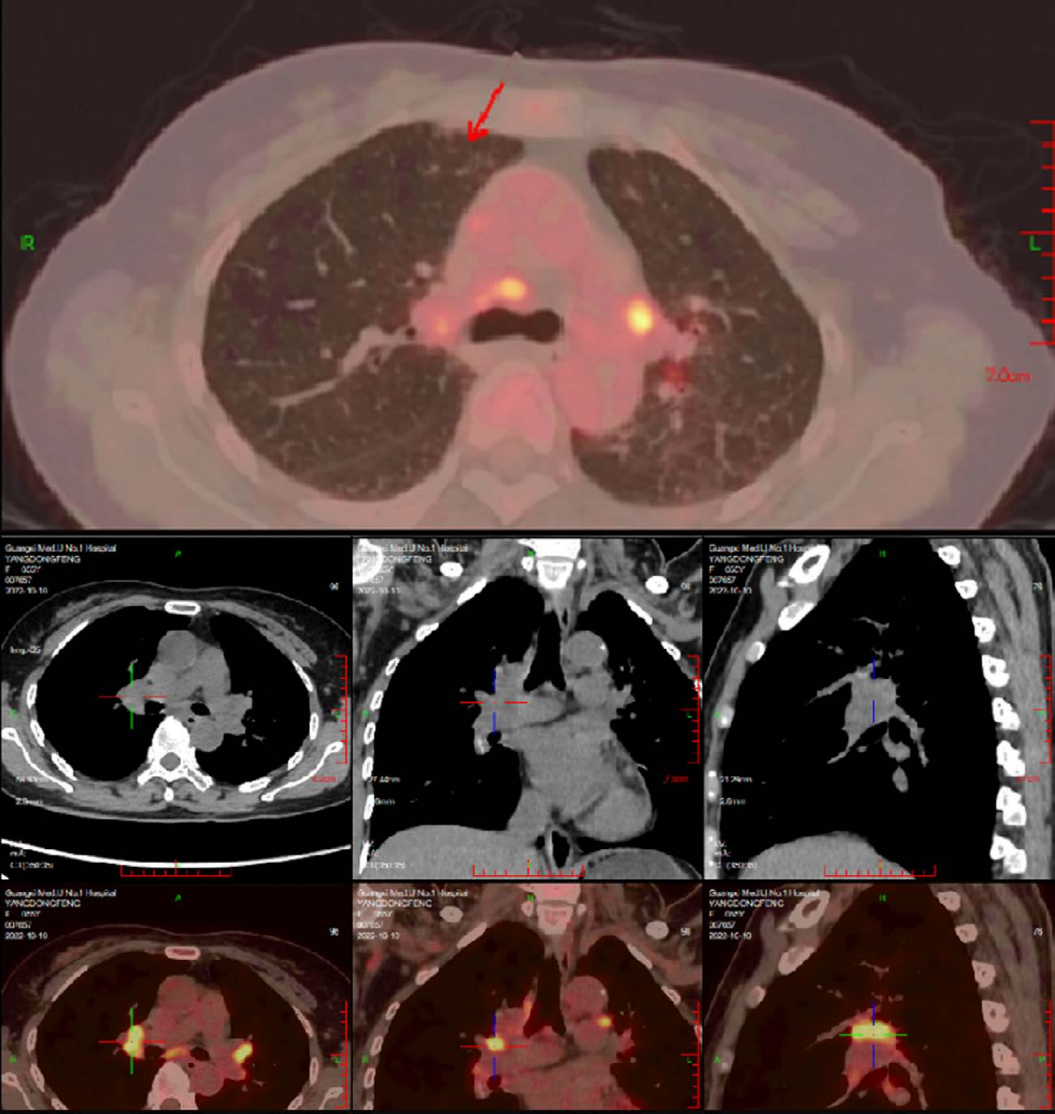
PET/CT images of the patient. Arrows showed inflammatory hyperplasia of multiple systemic lymph nodes (bilateral neck areas I, II, and V, bilateral axillae, mediastinum, bilateral hilum, and bilateral groin) and a slight inflammation in both lungs. PET/CT = positron emission tomography-computed tomography.
